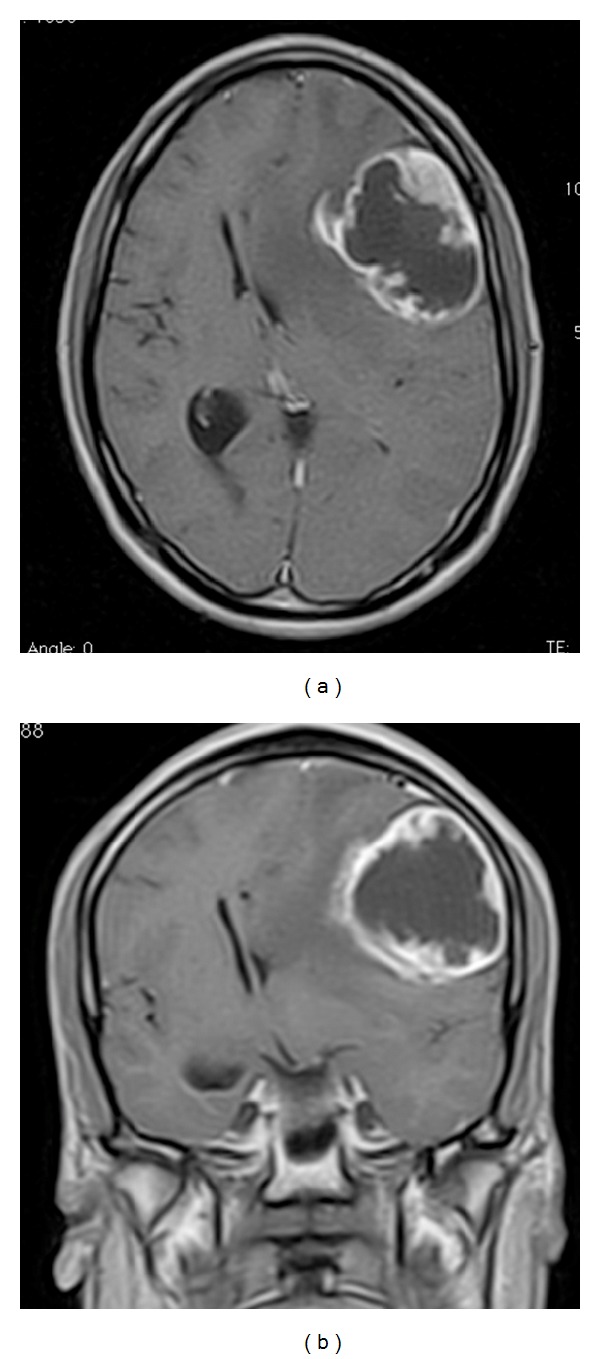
Preoperative MRI in axial (a) and coronal (b) view, showing extraventricular intraxial extensive lesion.
